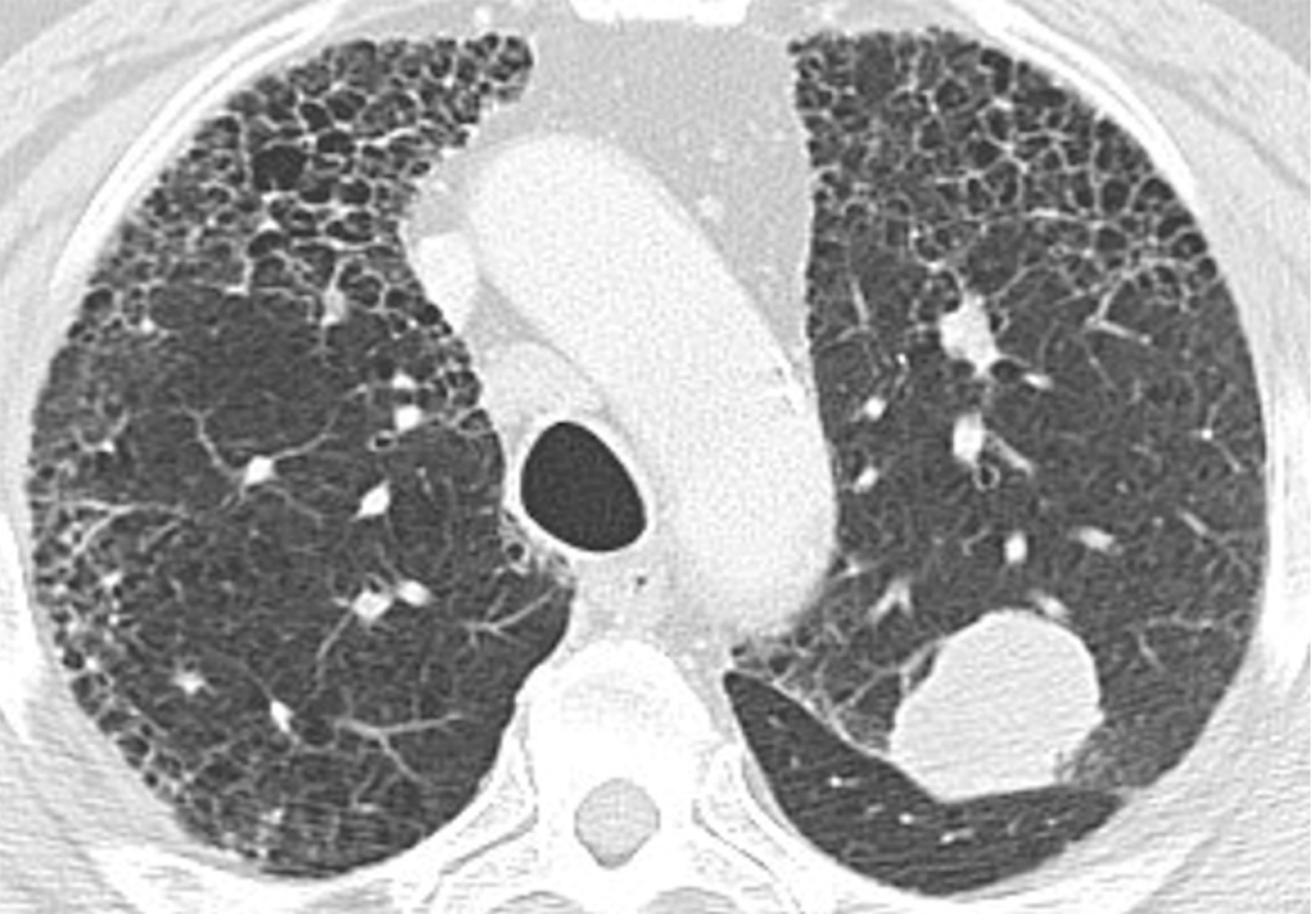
Squamous cell carcinoma and CT pattern typical for UIP. Axial CT image at the level of the upper lobes shows a left upper lobe squamous cell carcinoma and CT features of typical UIP.
